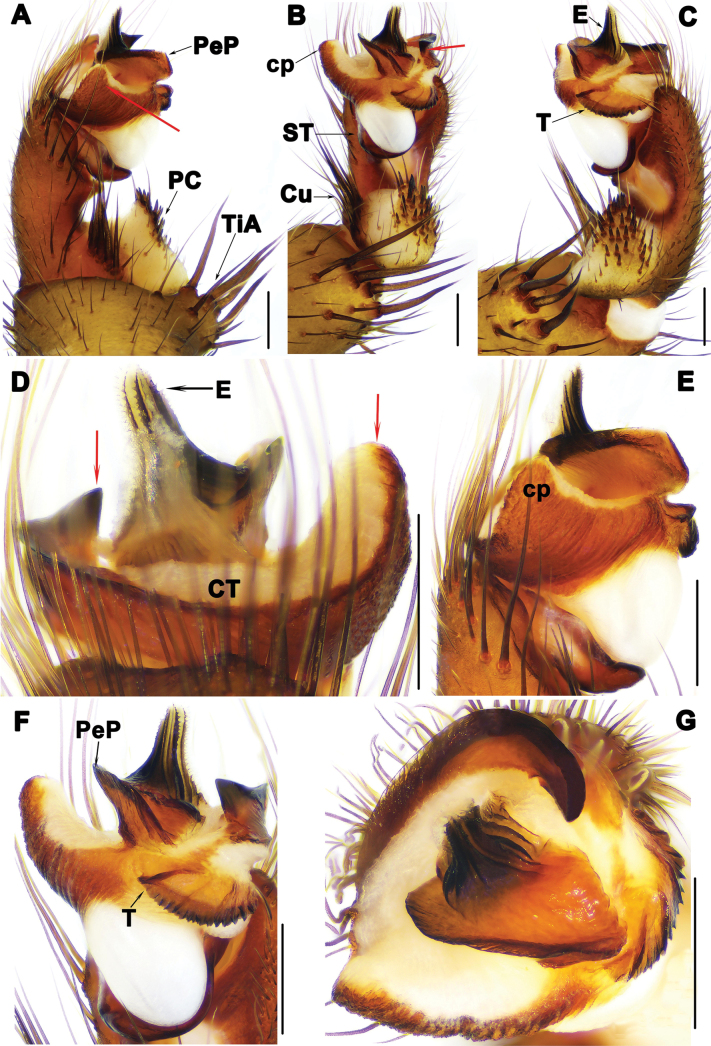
Male palp of *Liphistiuskalaw* sp. nov. A, E prolateral view B ventral view C, F retrolateral view D dorsal view, red arrows show arched projection and triangular process of CTG distal view. Abbreviations: CT = contrategulum; cp = contrategular process; Cu = cumulus; E = embolus; PC = paracymbium; PeP = paraembolic plate; ST = subtegulum; T = tegulum; TiA = tibial apophysis. Scale bars: 0.5 mm.
